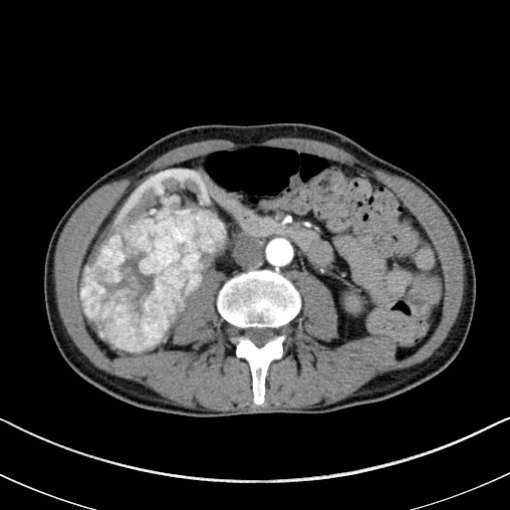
CT scan showing large tumor of the right kidney.
